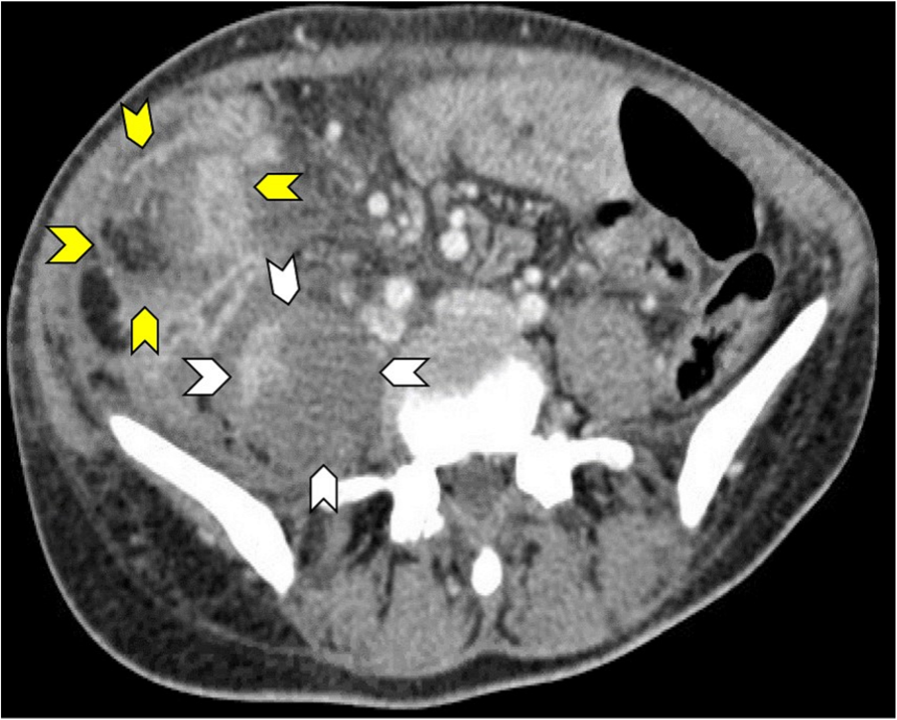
A 31-year-old woman presented with right iliac fossa and right-sided back pain for 1-month duration. She was afebrile, had no cough or night sweats but was anemic. Abdominal CT scan with intravenous contrast showed an inflammatory mass in the right iliac fossa (yellow arrow heads) with sinus tracts communicating to a right iliopsoas abscess (white arrow heads). The patient was from an endemic area of tuberculosis and was treated with anti-tuberculous drugs
